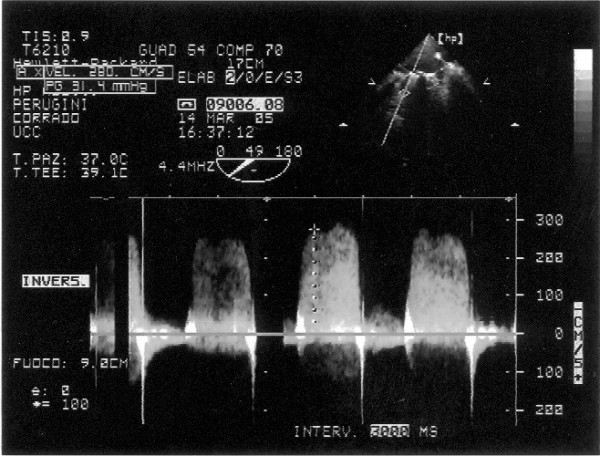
Transesophageal echocardiography: Doppler CW on mitral prosthesis valve with the evidence of transvalvular gradient.
